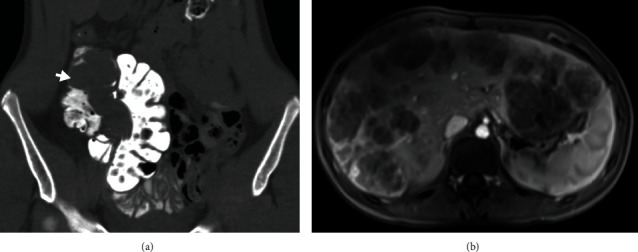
(a) Coronal CT image of the abdomen and pelvis with oral contrast demonstrates an intraluminal mass in the terminal ileum and ascending colon (arrow) serving as a lead point for ileocolic intussusception. (b) Axial T1 fat-saturated postcontrast MRI of the abdomen demonstrates multiple peripherally enhancing lesions throughout the liver consistent with liver metastasis.
